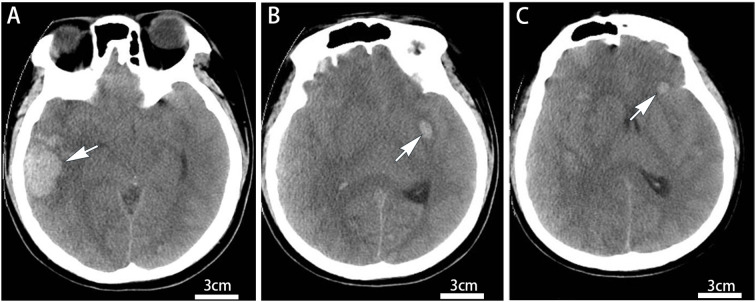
CT shows acute intracranial hemorrhages. Axial non-contrast images (A–C): (A) right temporal lobe, (B) left basal ganglia, (C) left frontal lobe. Scale bar = 3 cm; window width 100 HU, level 35 HU. Hemorrhage volumes (ABC/2 method): (A) 17.6 ml, (B) 1.1 ml, (C) 0.3 ml. White arrows indicate hemorrhages.
